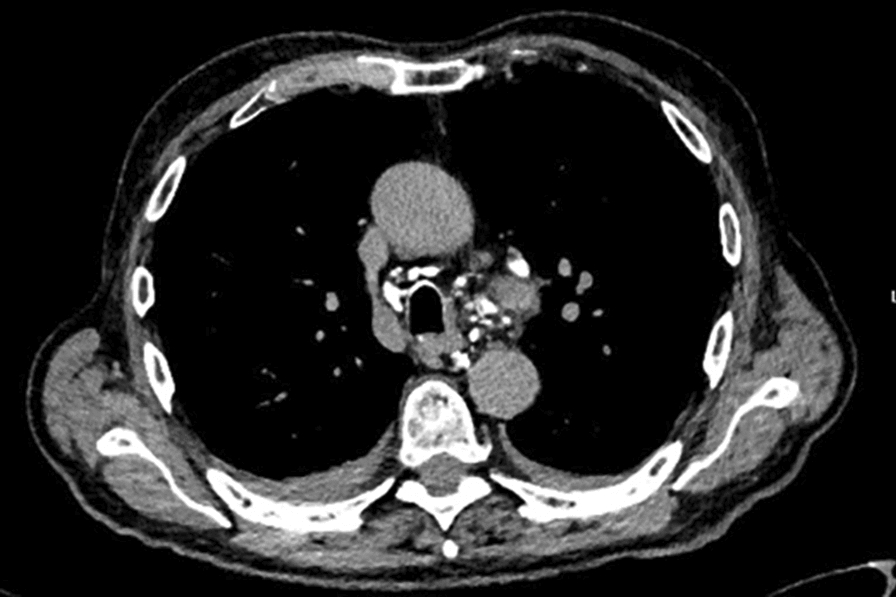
CT lymphangiography—transversal view, caudal to the mediastinal lipoma
